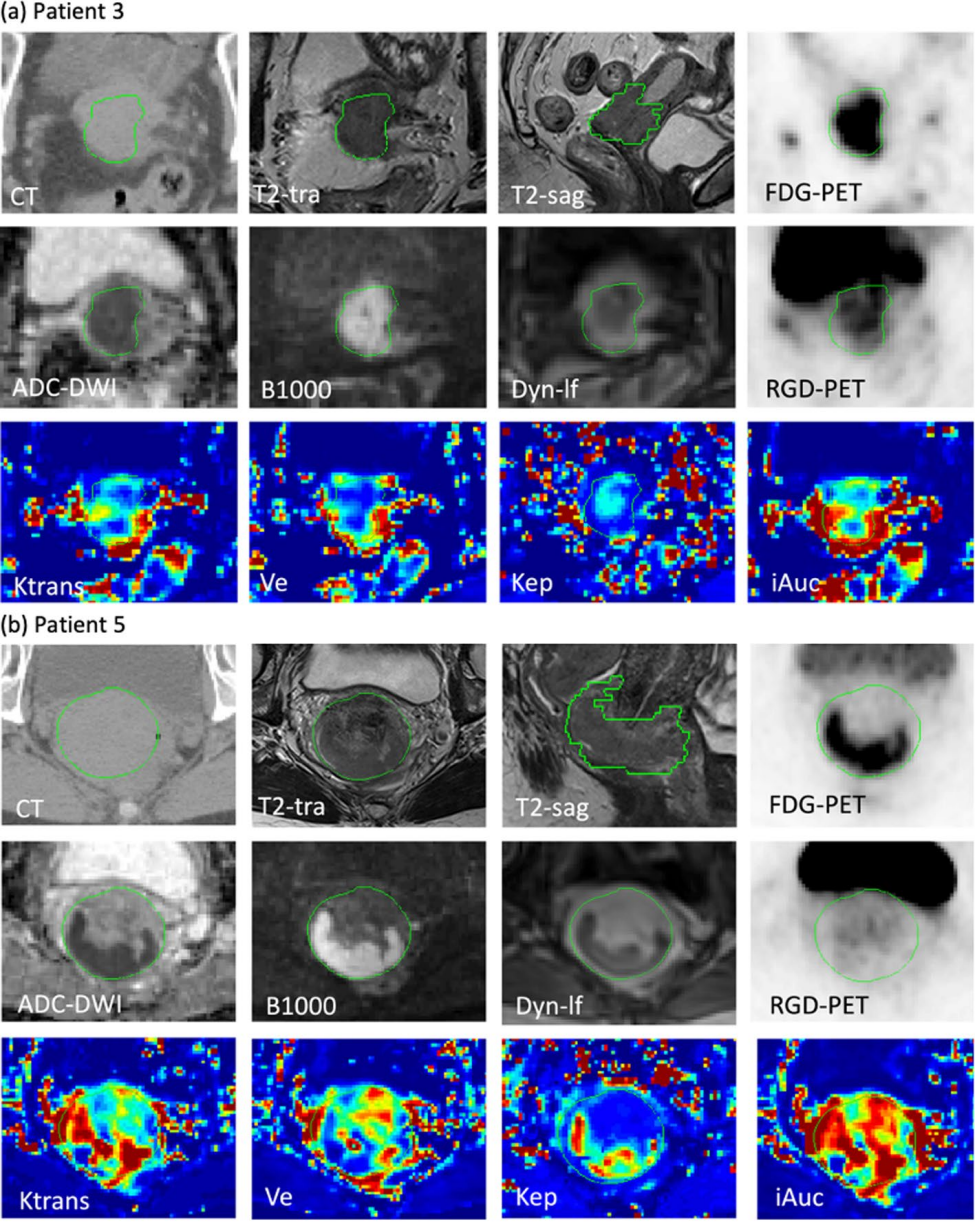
Representative examples of co-registered parametric maps for patient 3 (a) and patient 5 (b). From upper left to lower right: planning CT, axial and sagittal T2-weighted MRI, FDG-PET, diffusion parameters (ADC, B1000), last frame of DCE, RGD-PET, and perfusion parameters (*K*^trans^, *v*_e_, *k*_ep_, and iAUC_60_). Green contour represents GTV
